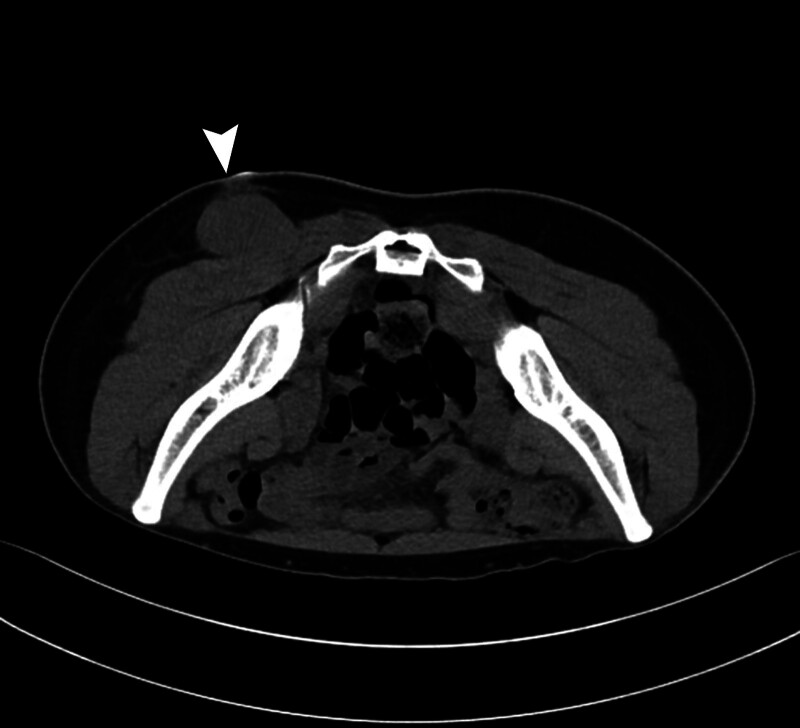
Initial CT imaging showing a 4.5-cm ovoid, dense soft tissue mass at the posterior aspect of the left gluteus maximus muscle, with indistinct borders. CT = computed tomography.
